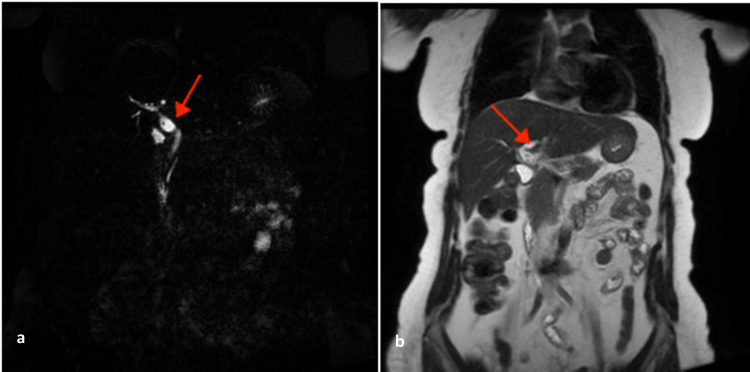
MRI with sequence heavily weighted in T2 (a) and coronal slice in T2 (b) showing segmental dilation of the main bile duct (red arrow) with endoluminal lithiasis. MRI: magnetic resonance imaging.
